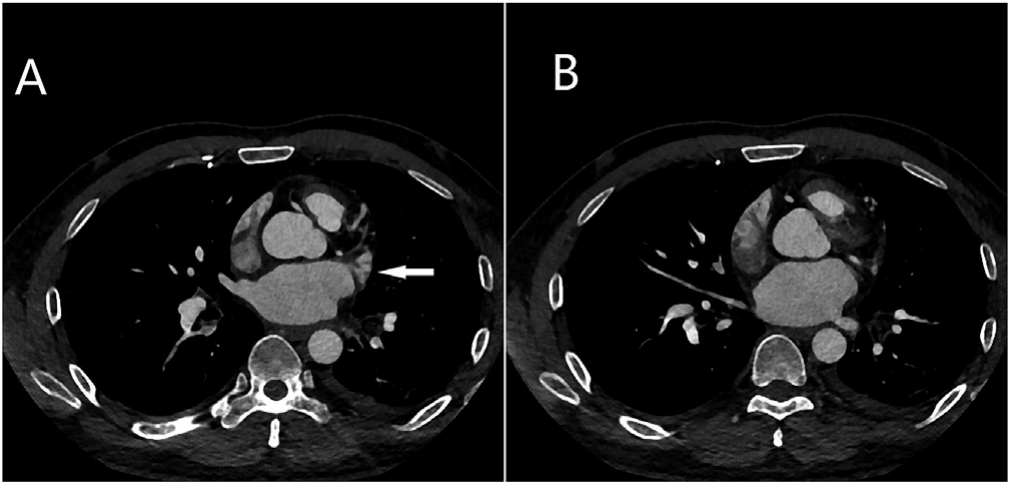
Pulmonary vein CT angiography scan showing multiple filling defects at the proximal end of the left superior pulmonary vein, representing thrombosis (A); The distal trunk and branches of the left superior pulmonary vein are completely occluded (B). CT: computed tomography.
